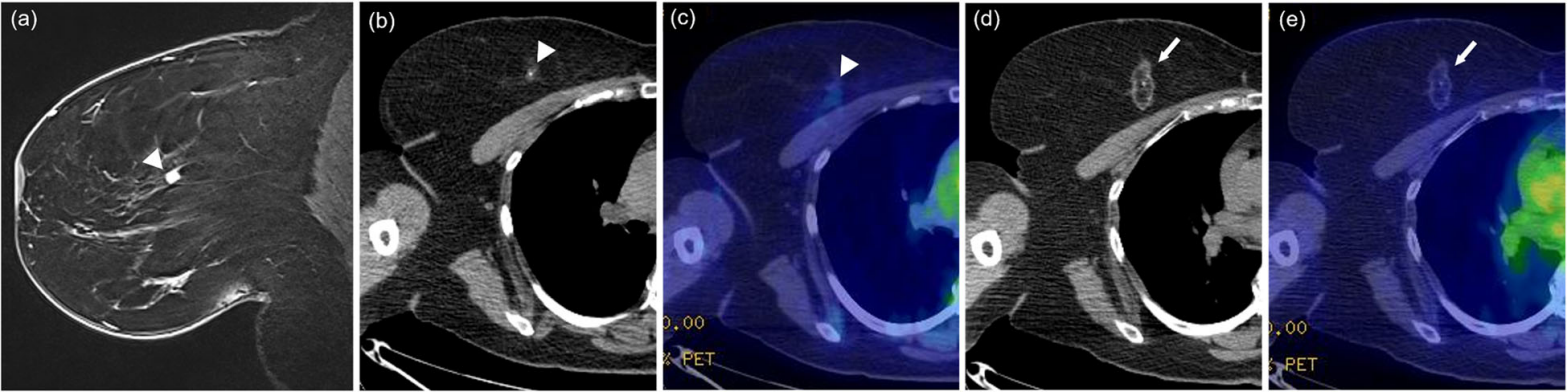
Fatty mass type. A 55-year-old woman with invasive ductal carcinoma. Breast magnetic resonance imaging (MRI) before cryoablation (a, arrowhead) showing a solid nodule of diameter 8 mm in the inner-upper quadrant of the right breast. The pre-cryoablation CT part of PET/CT (b, arrowhead) does not clearly show the breast cancer lesion identified by MRI. The pre-cryoablation fusion image of PET/CT (c, arrowhead) shows subtle FDG uptake (SUVmax, 0.89). On the CT portion (d, arrow) and (e, arrow) of the fusion image of PET/CT that was obtained 36 months after cryoablation, the treated area shows a focal fatty area surrounded by a rim like soft tissue density with subtle FDG uptake (SUVmax, 0.82). The calcification at the center of the treated area was present prior to cryoablation
